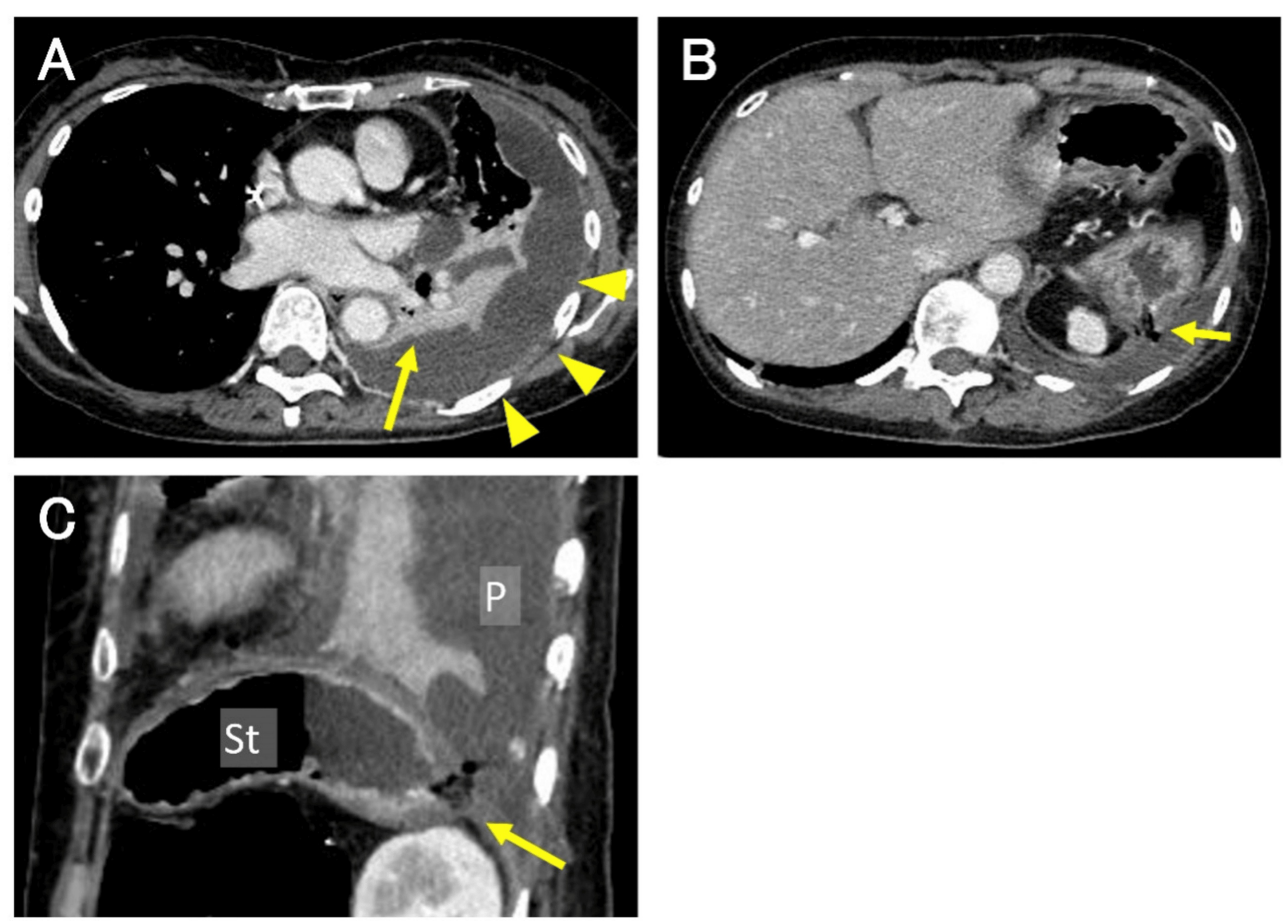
Contrast-enhanced computed tomography (A) Axial view of contrast-enhanced computed tomography (CECT) showing a significant fluid collection in the left pleural cavity (arrowheads) and left basilar atelectasis (arrow) (B) Axial view of CECT suggesting a fistula between the stomach and the left pleural cavity, corresponding to the location of the peritoneal metastasis at the left subdiaphragm (arrow) (C) Sagittal view of CECT demonstrating the fistula (arrow) St: stomach, P: pleural cavity
